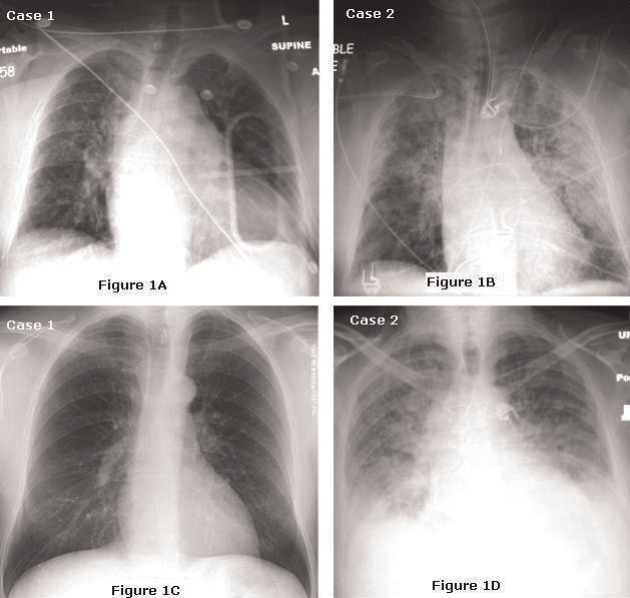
CXR findings in GP IIb/IIIa induced DAH. (A & B) Anteroposterior view of chest x-ray at presentation. (C) Anteroposterior view of chest x-ray seven days later. (D) Anteroposterior view of chest x-ray day later.
